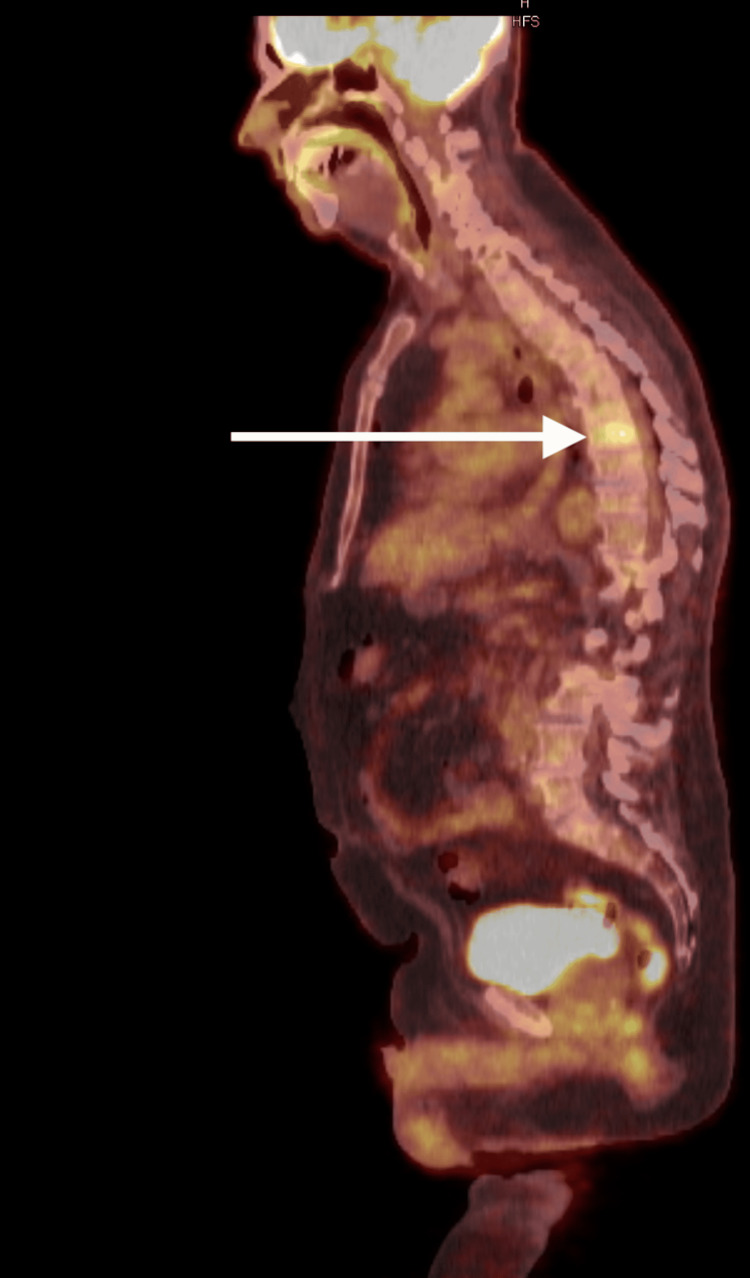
PET/CT showing osseous lytic lesion in T8 vertebra by white arrow
